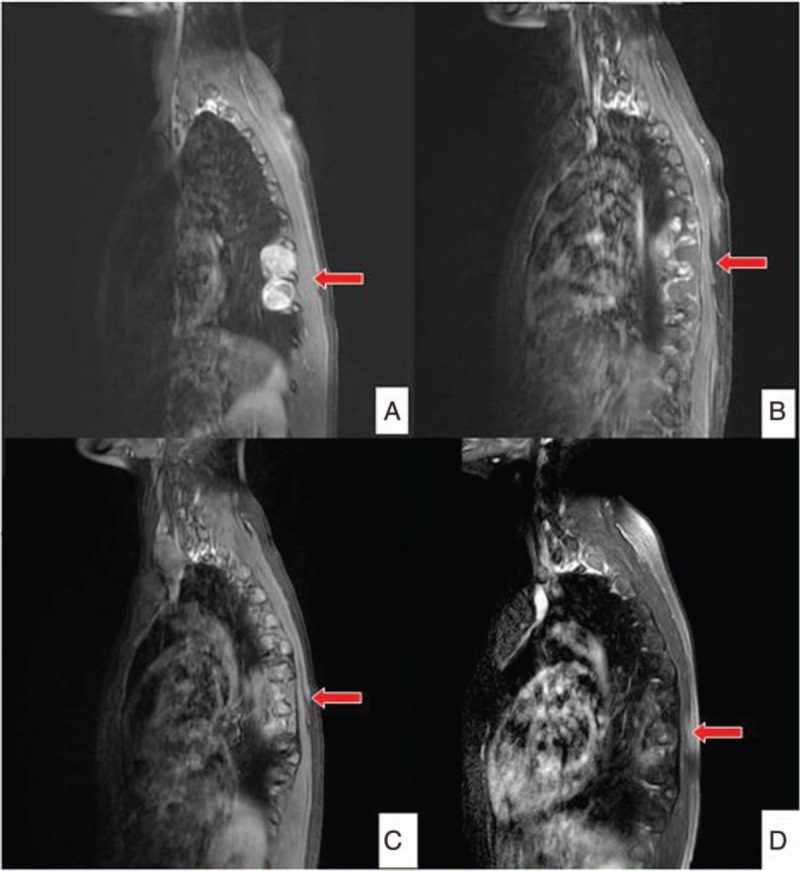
Serial images of T-spine magnetic resonance imaging. (A) T-spine tumor first noted in 2009. (B) After first tumor removal. (C) Recurrence of T-spine tumor. (D) Follow-up image after second operation in 2015 (red arrows indicate the lesions).
